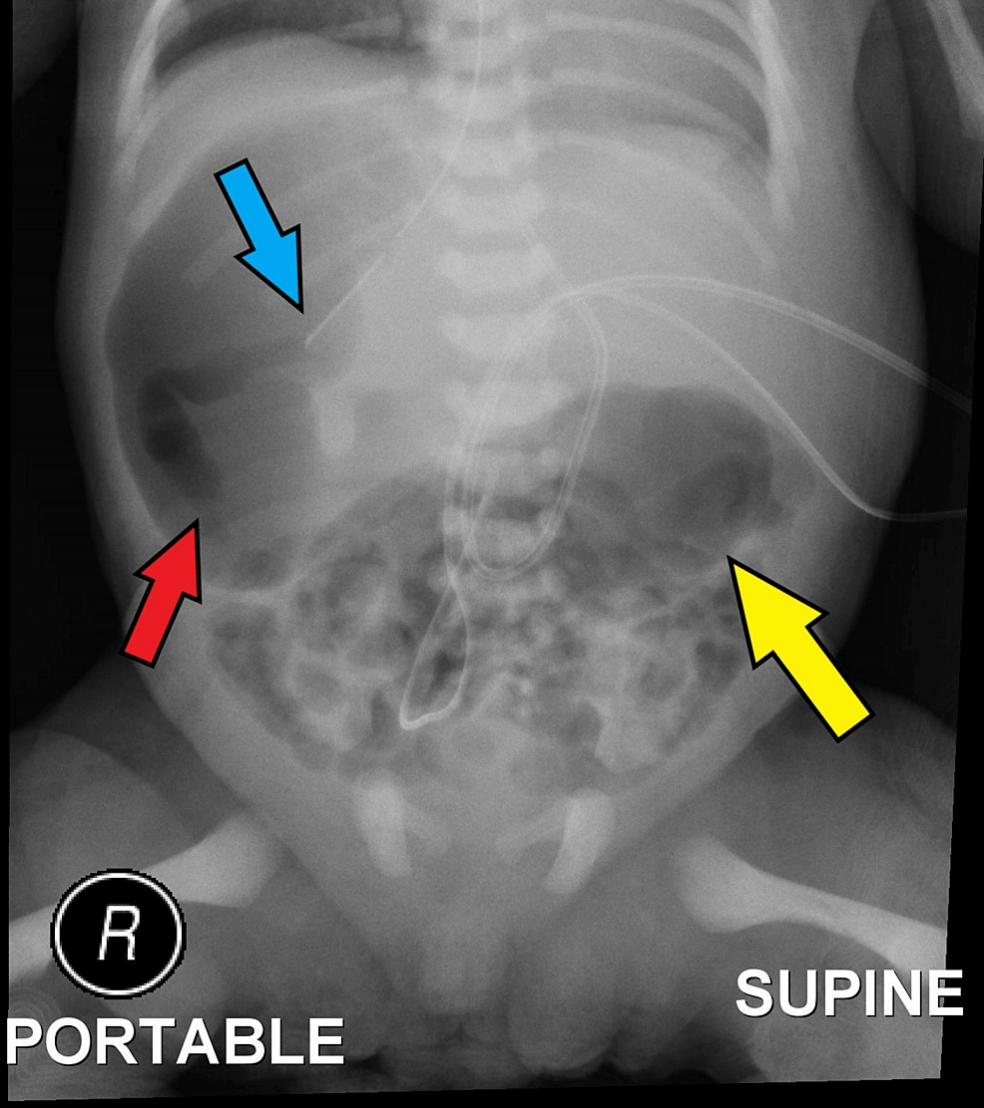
Abdominal X-ray of the patient showing the tip of the feeding tube in the right hypochondrium (blue arrow), dilated bowel gas over the right hypochondrium (red arrow), and dilated bowel gas central abdomen (yellow arrow).
